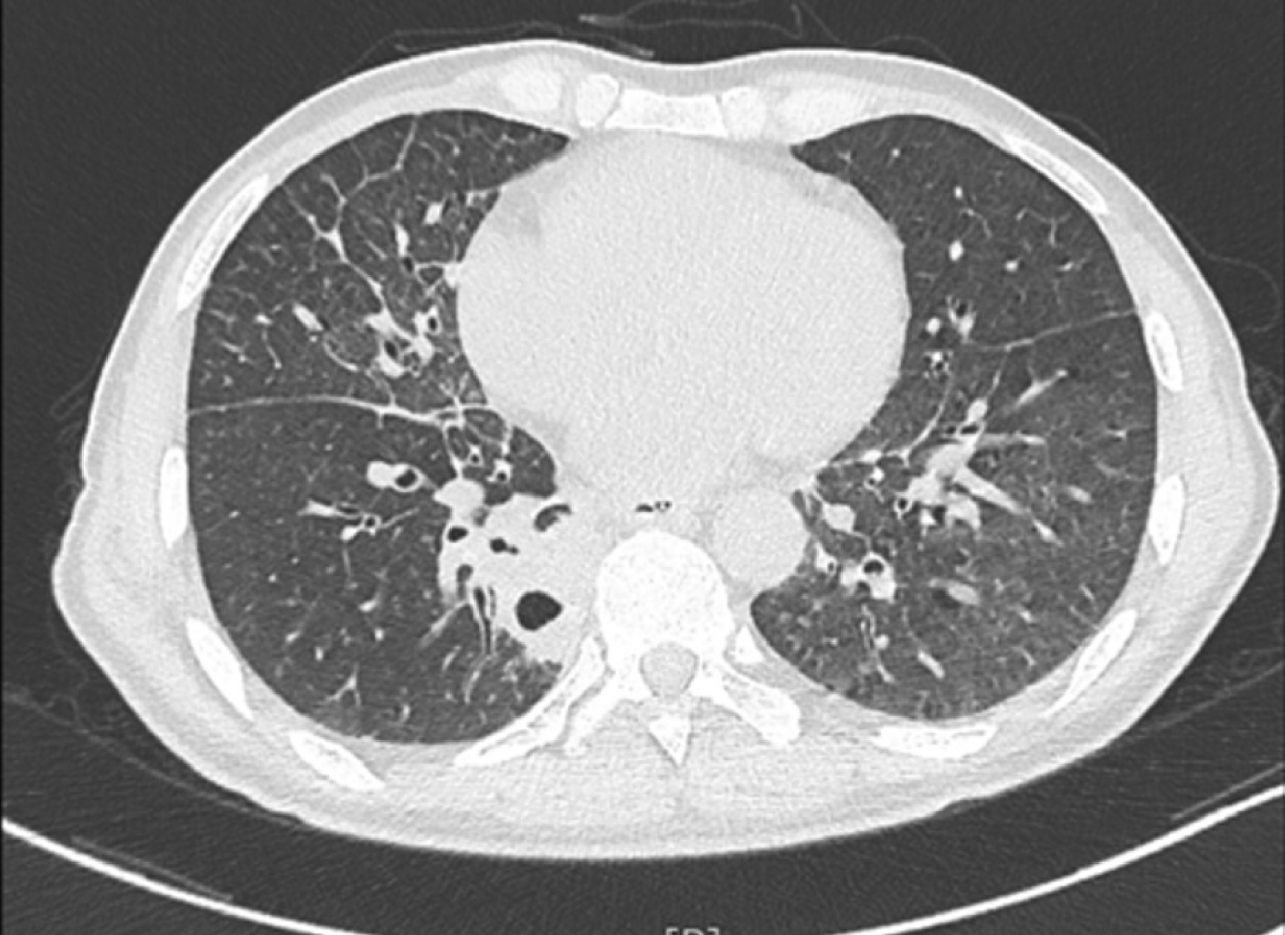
Computed tomography (CT) demonstrated cavitations, consolidations and mass, consistent with known CT manifestations of typical mucormycosis.
